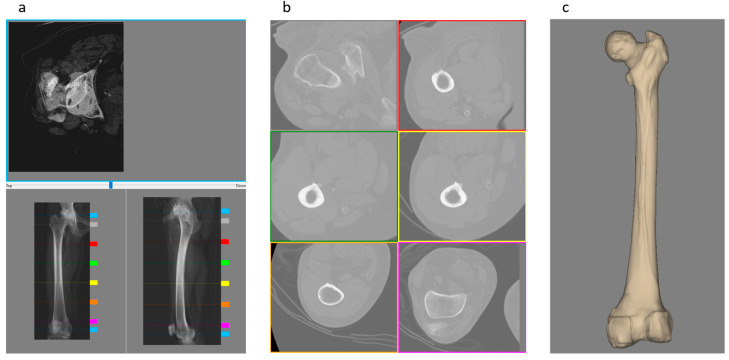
The multimodal display principles. (a) The XR-window where the CT dataset is represented as two orthogonal radiography projections: antero-posterior view (bottom left) and medio-lateral view (bottom right), together with a merged view (top) of two CT slices to visualize the femoral anteversion. The colored sliders at the edge of the XR-projection image are used to choose the CT slices for the anteversion representation (blue sliders) and (b) the six CT slices of the CT-window (sliders from gray to magenta); (c) the 3D-window, where the femoral bone is represented with a surface rendering.
